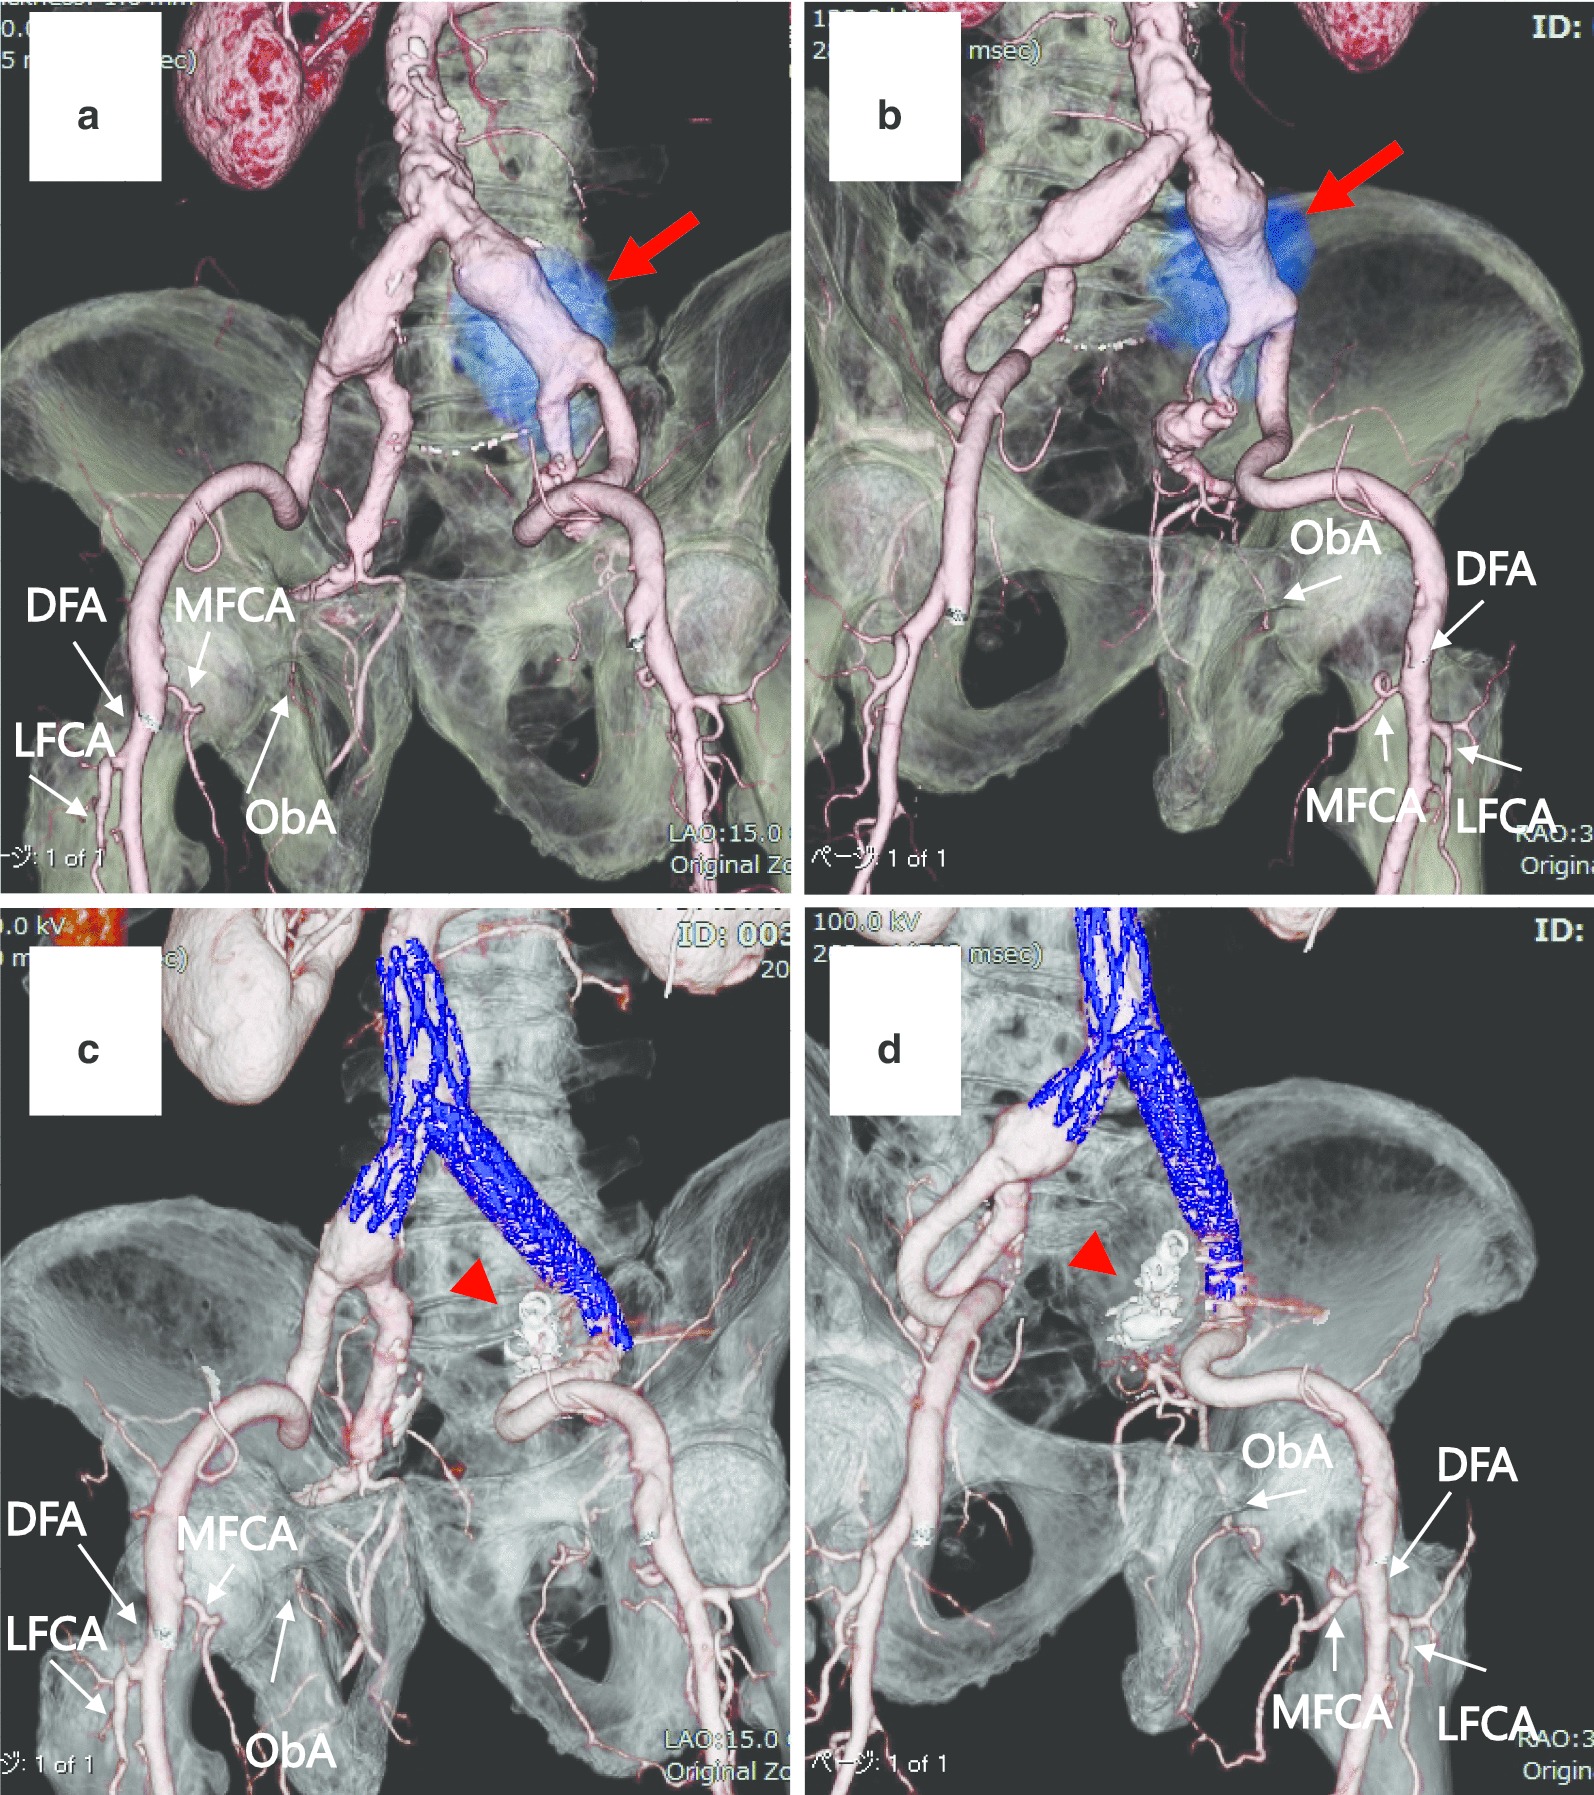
3DCTA in a case of isolated CIA aneurysm. The bilateral superficial femoral arteries were deleted in the all Figures. a Preoperative 3DCTA showing an isolated left CIA aneurysm (red arrow), the contralateral DFA, LFCA, MFCA and ObA. b Preoperative 3DCTA showing an isolated left CIA aneurysm (red arrow), the ipsilateral DFA, LFCA, MFCA and ObA. c Postoperative 3DCTA showing the exclusion of the left CIA aneurysm using a stent graft with left IIA interruption (arrowhead), the contralateral DFA, LFCA, MFCA and ObA. d Postoperative 3DCTA showing the exclusion of the left CIA aneurysm using a stent graft with left IIA interruption (arrowhead), the ipsilateral DFA, LFCA, MFCA and ObA, and the ipsilateral MFCA communicated with ipsilateral ObA. *3DCTA* three-dimensional contrast-enhanced computed tomography angiography, *CIA* common iliac artery, *DFA* deep femoral artery, *LFCA* lateral femoral artery, *MFCA* medial femoral circumflex artery, *ObA* obturator artery, *IIA* internal iliac artery
